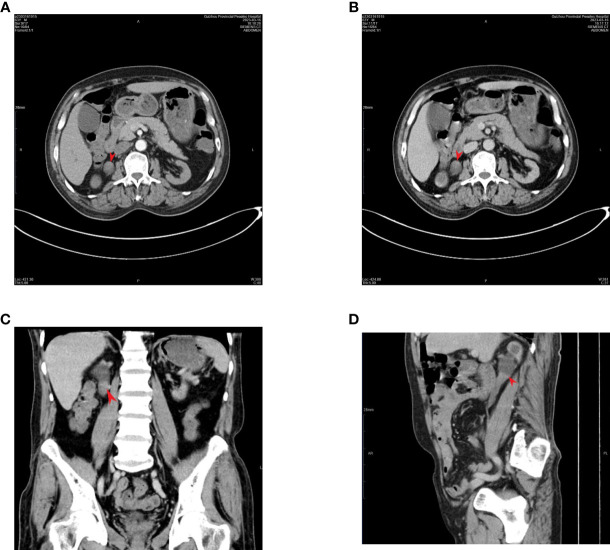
Enhanced CT of the urinary system: a soft tissue mass is visible within the right superior ureter, with mild enhancement noted on an enhanced scan. (tumor location flat against the 2nd-3rd lumbar vertebrae). The arrow points to the tumor: (A) arterial phase; (B) venous phase; (C) coronal plane; (D) sagittal plane.
